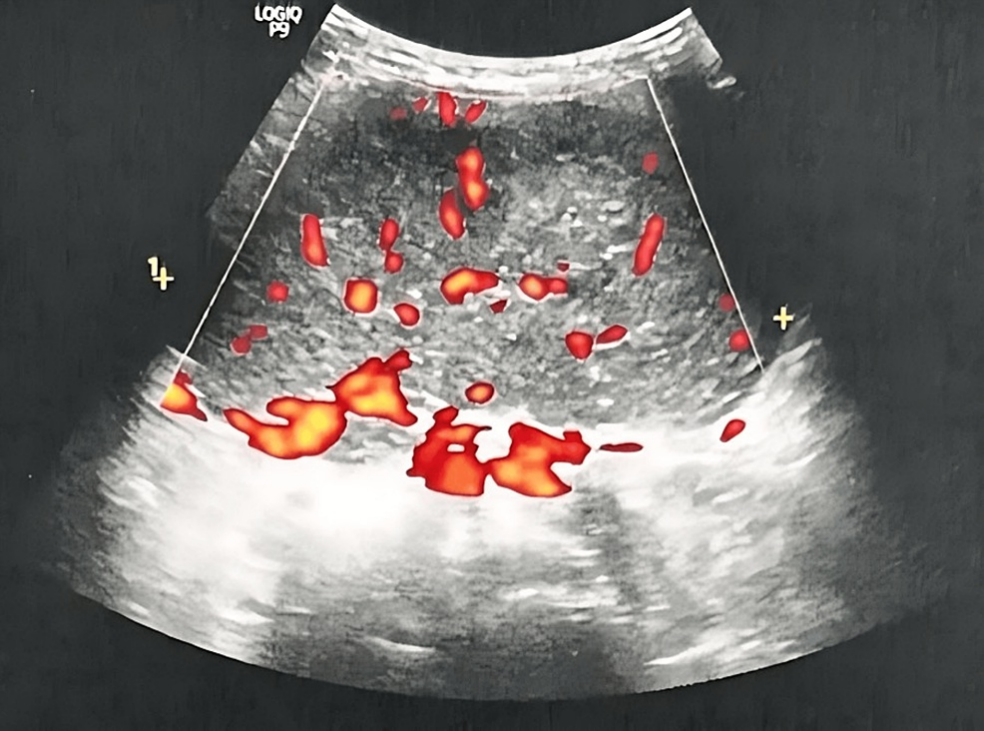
Pelvic ultrasound revealed an 11 x 6 cm median solid lesion more developed on the right side.
